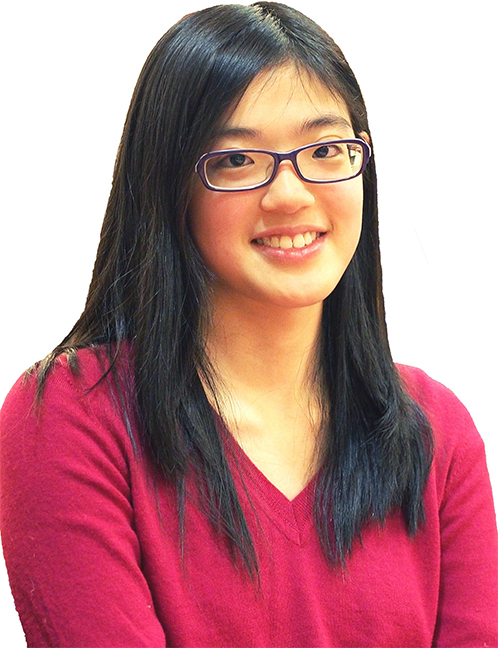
Dr Lanuti. Thank you. You mentioned that you observed an ablation margin of about 6 mm. When we do transthoracic thermal ablation, we generally try to reach 10 mm. Can you tell us, is there a limitation of the delivery or have you lowered your standard for margin?
